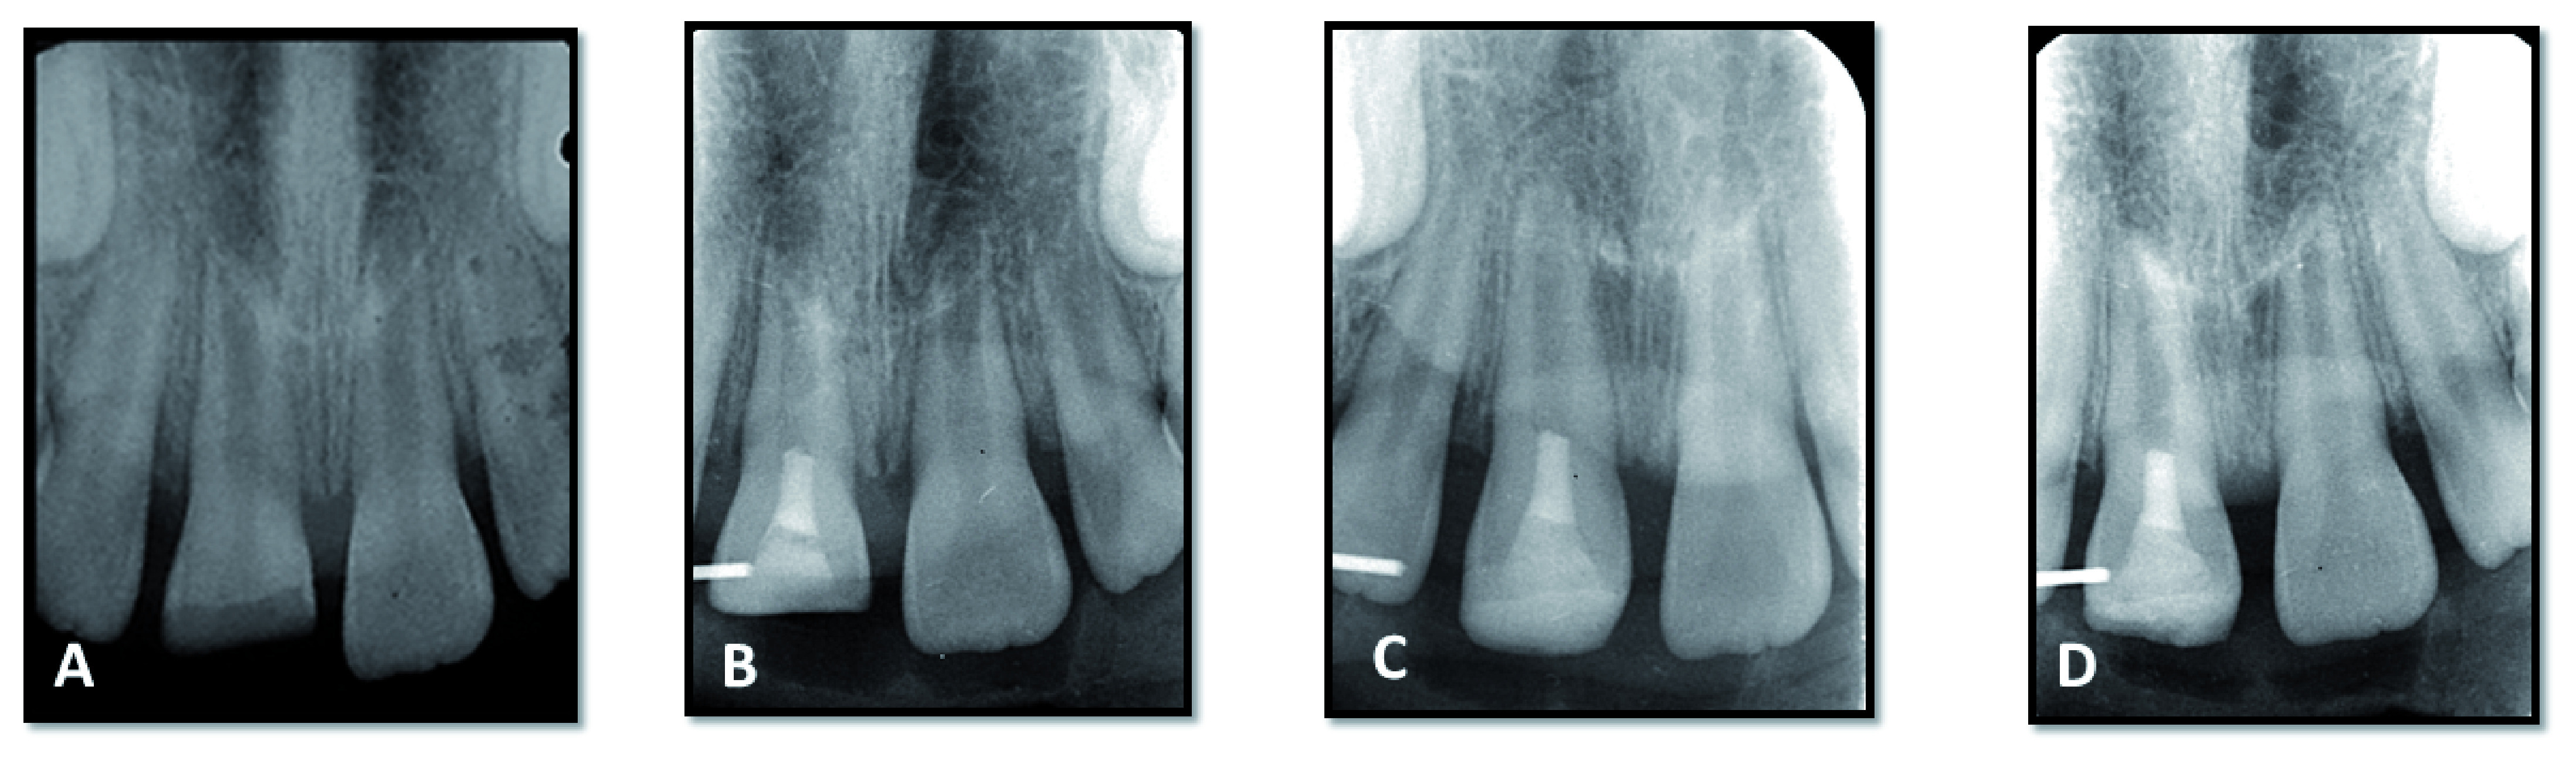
A) preoperative periapical radiograph;
